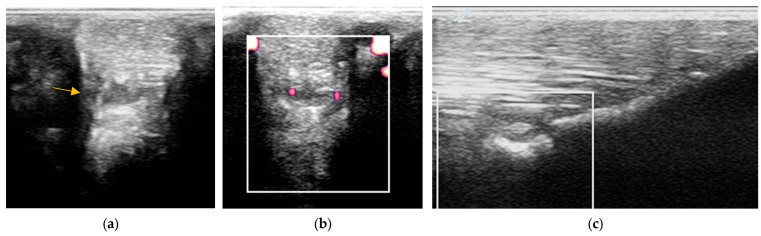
The same suspensory ligament branch as Figure 8, acquired four years after the initial injury. (a) Transverse image at the mid region, (b) transverse power Doppler image at the same level as (a,c) longitudinal power Doppler image of the distal region (proximal is to the left). (a) There is a new injury at a more proximal site (arrow) than the original injury, with mild Doppler activity (b). At the original injury site (c), a hypoechoic lesion is still apparent, but no Doppler signal was detected. There was localised swelling at the injury site, and distension of the metacarpophalangeal joint capsule.
